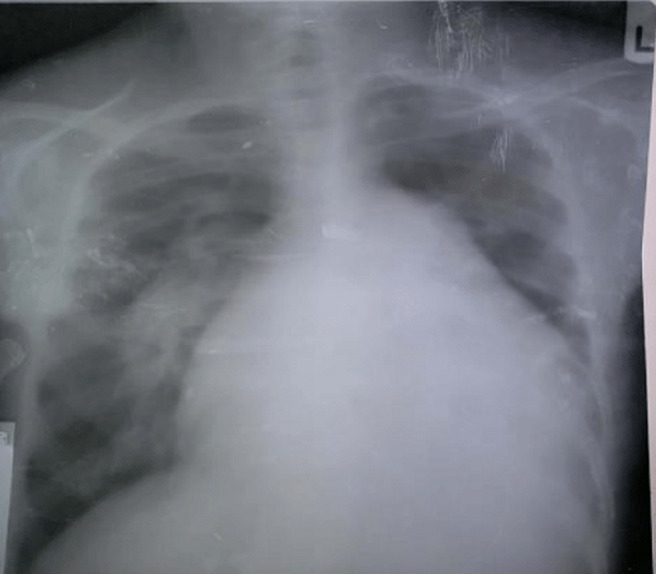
chest X-ray P/A view showing bulged pulmonary conus, cardiomegaly with CTR (cardiothoracic ratio) of 0.8 and pulmonary plethora
